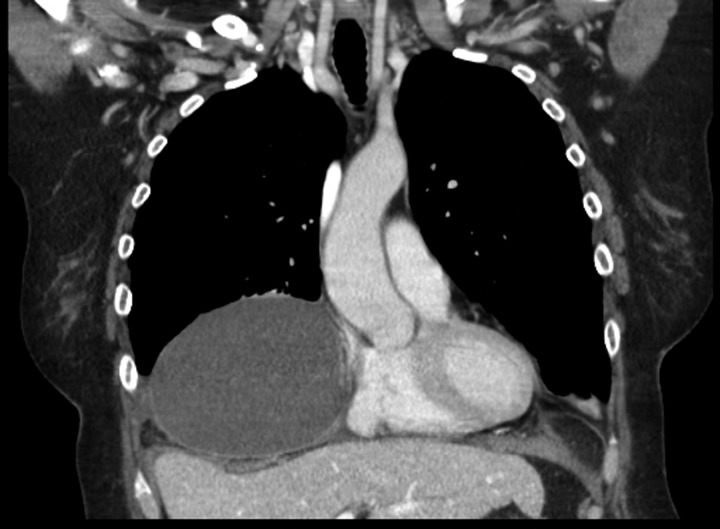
One month postoperative CT chest revealing a large seroma had formed in the area of the previous hernia.
